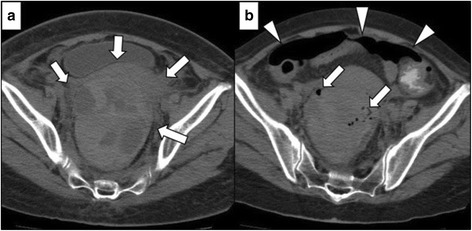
Computed tomography (CT) findings. a Rectal hematoma (*arrows*) b Intraperitoneal free air (*arrow heads*) and bubbles in the rectal hematoma (*arrows*)
